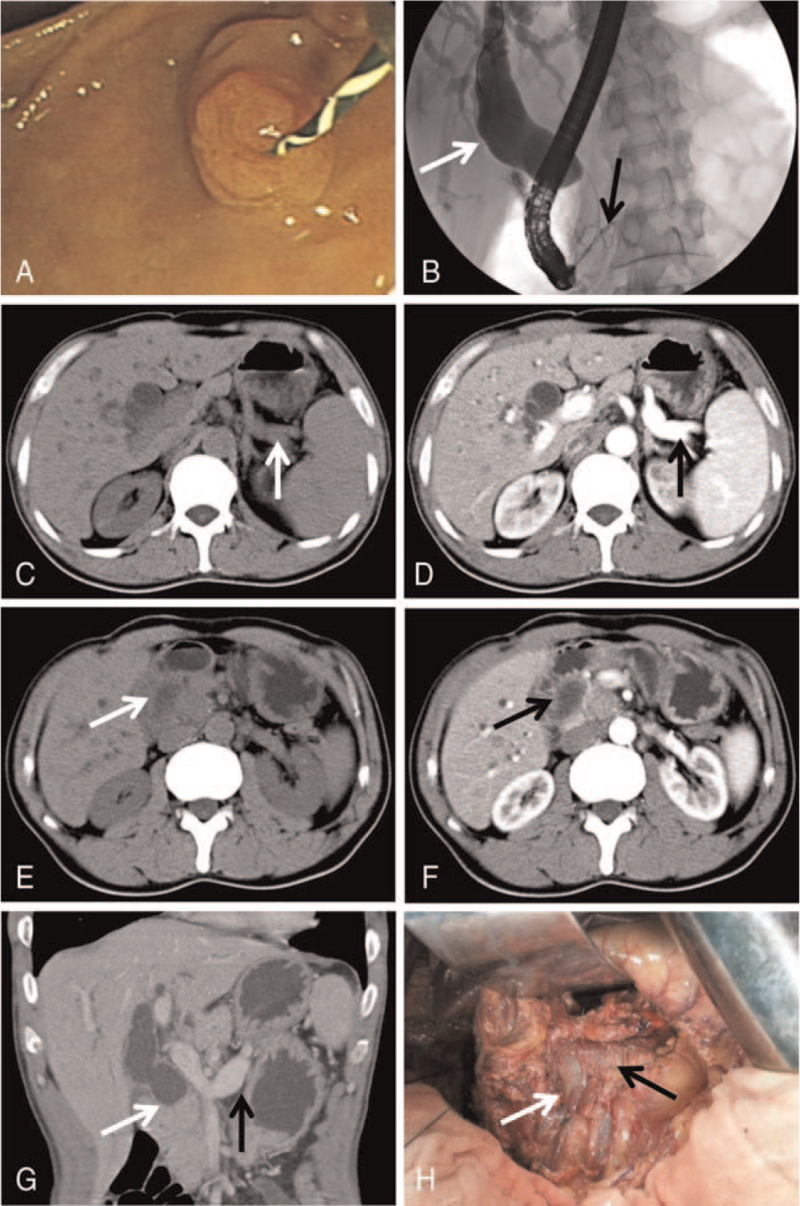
Endoscopic retrograde cholangiopancreatography (ERCP) images, abdominal CT imaging and intraoperative image of the reported ADP case. (A) Major duodenal papilla by duodenoscopy. (B) ERCP showing dilated common bile duct and the location of the bile duct obstruction (white arrow), and the pancreatic duct located at the head of the pancreas (black arrow). Non-contrast CT (C) and contrast-enhanced CT (D) showed splenic vessels (C, white arrow; D, black arrow) and complete absence of the body and tail of pancreas. Non-contrast CT (E) and contrast-enhanced CT (F) showed a mass located at pancreas head with dilation of common bile duct (E, white arrow; F black arrow). Coronal CT scanning (G) showed the pancreas head and dilated common bile duct (white arrow), and the splenic vein with absence of the body and tail of pancreas (black arrow). Intraoperative image (H) showed superior mesenteric vein (white arrow), and the splenic vein with absence of the body and tail of pancreas (black arrow).
